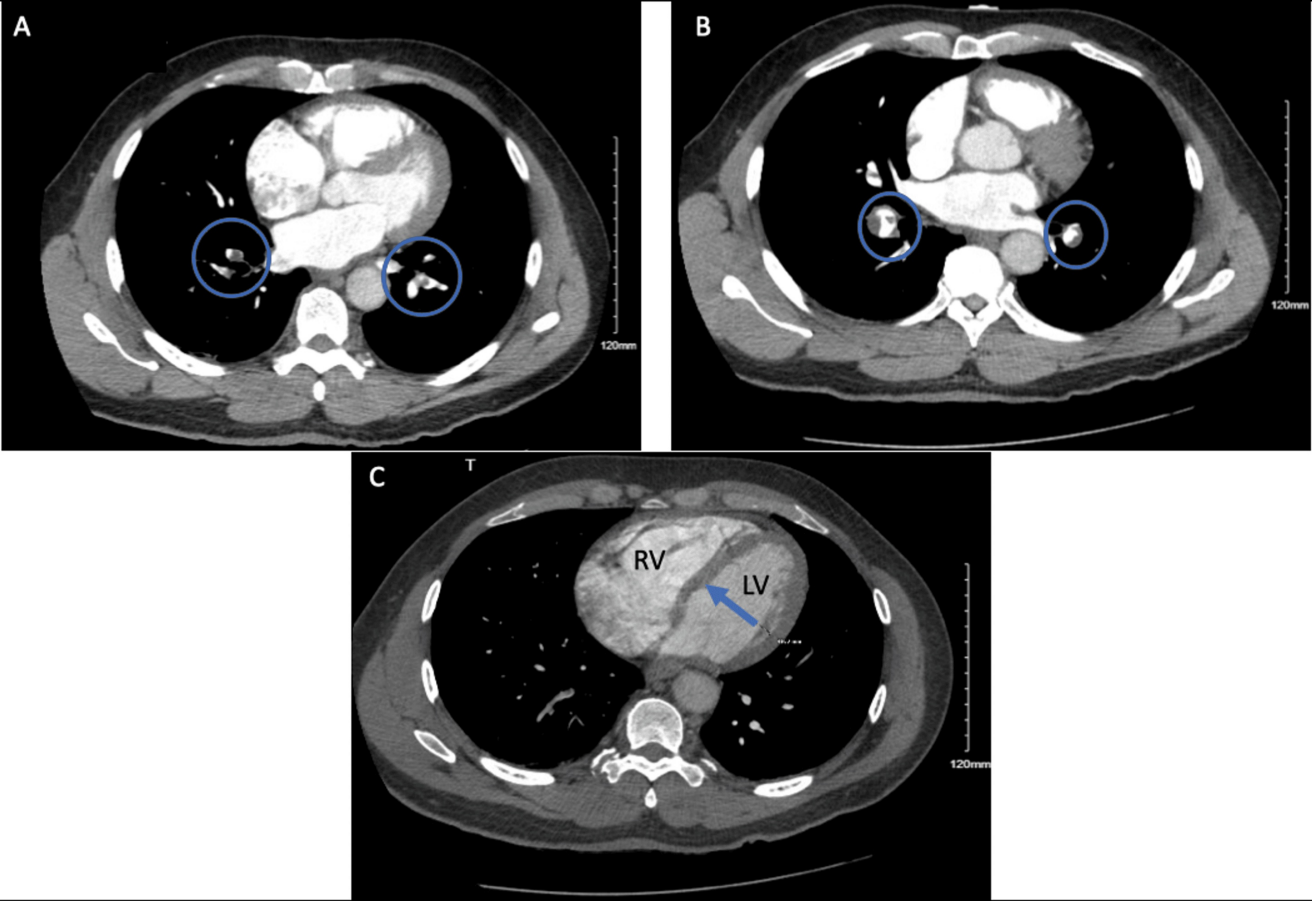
CTA showing bilateral filling defects signifying segmental and lobar pulmonary emboli (blue circles) in images A and B. Image C shows a flattened interventricular septum (blue arrow) with a relatively enlarged right ventricle signifying CTA evidence of right heart strain. CTA, computed tomography with angiography LV, left ventricle; RV, right ventricle
